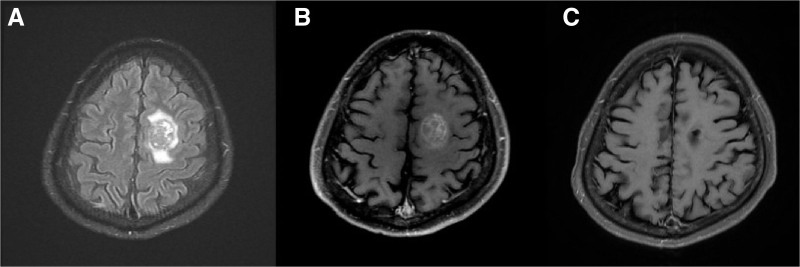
Representative magnetic resonance images of patient with long-term response to dendritic cell vaccination. Brain MRI images of Patient 1, a 36-yr-old man with brain metastasis from lung adenocarcinoma. (A) Preoperative T2 FLAIR image showing left parietal tumor with surrounding edema. (B) Preoperative T1 contrast-enhanced image demonstrating enhancing left parietal lesion. (C) T1 contrast-enhanced image 22 mo postsurgical resection and completion of dendritic cell vaccination, showing no evidence of tumor recurrence. This patient survived 51 mo from diagnosis despite having stage IV disease. FLAIR = fluid-attenuated inversion recovery, MRI = magnetic resonance imaging.
